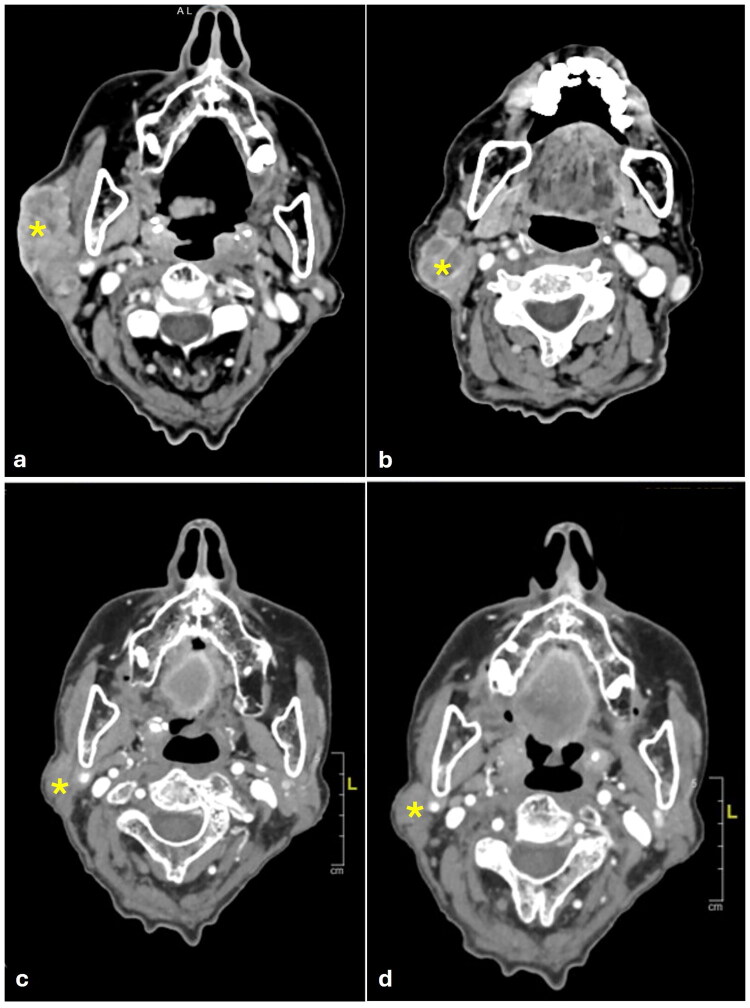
a–d: Head and neck CT scan. a) The yellow asterisk points an lesion with heterologous tissue components inseparable from a colliquative lymphadenopathy package for a maximum overall extension of 80 × 80 × 40 mm at the level of the right parotid lodge; b) The yellow asterisk points a rounded lymphadenopathy, approximately 8 mm in maximum diameter, at the right level IIB. c) The yellow asterisk points the thickening of the skin surface with modest retractive effects and obliteration of the adipose surface at the site of the lesion previously reported lesion in the right parotid lodge; d) The yellow asterisk points the thickening of the skin surface at the site of the previously reported lymphadenopathy in the IIB level.
